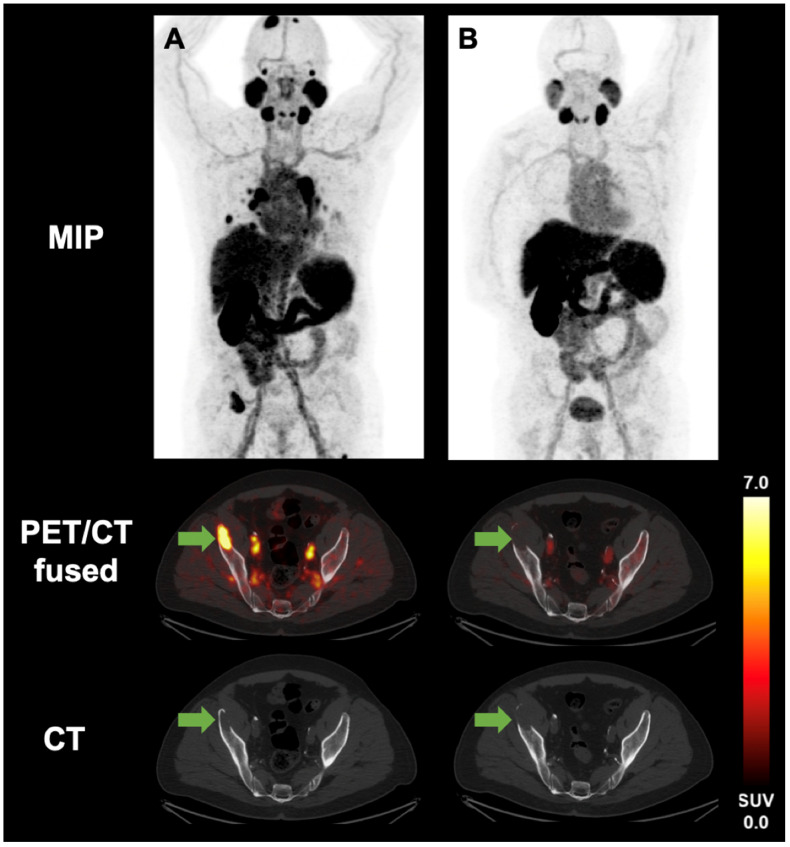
[^18^F]PSMA-1007 PET/CT of a 64-year-old male patient with metastatic ccRCC before (A) and after (B) therapy initiation. The arrow points to an osseous metastasis of the right iliac crest. Prior to systemic therapy (A) the SUV_max_ of the osseous metastasis was 11.0; after therapy initiation (B) no PSMA uptake was observed.
